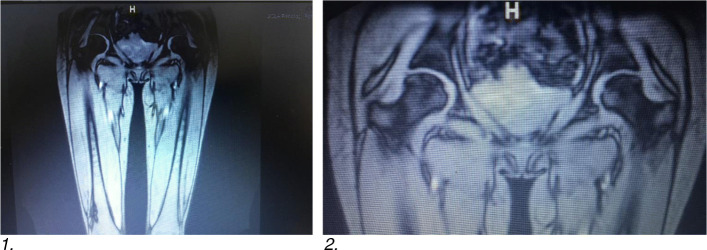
a MRI of bilateral lower extremities without contrast showing myositis of the quadriceps muscles. b Avascular necrosis of the femoral heads
